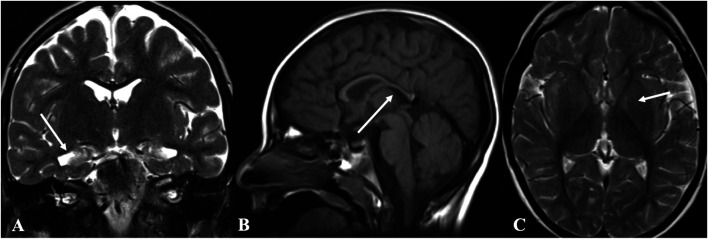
Brain magnetic resonance imaging (MRI) of patient 2. Brain MRI demonstrated temporal mesial bilateral hypotrophy (arrow in A, coronal T2w), especially affecting the hippocampus, aspecific white matter anomalies in peritrigonal and periventricular regions, diffuse supratentorial sulci enlargement, posterior corpus callosum thinning (arrow in B, sagittal T1w), and bilateral signal alterations in globus pallidus (arrow in C, axial T2w), substantia nigra, and dentate nucleus.
